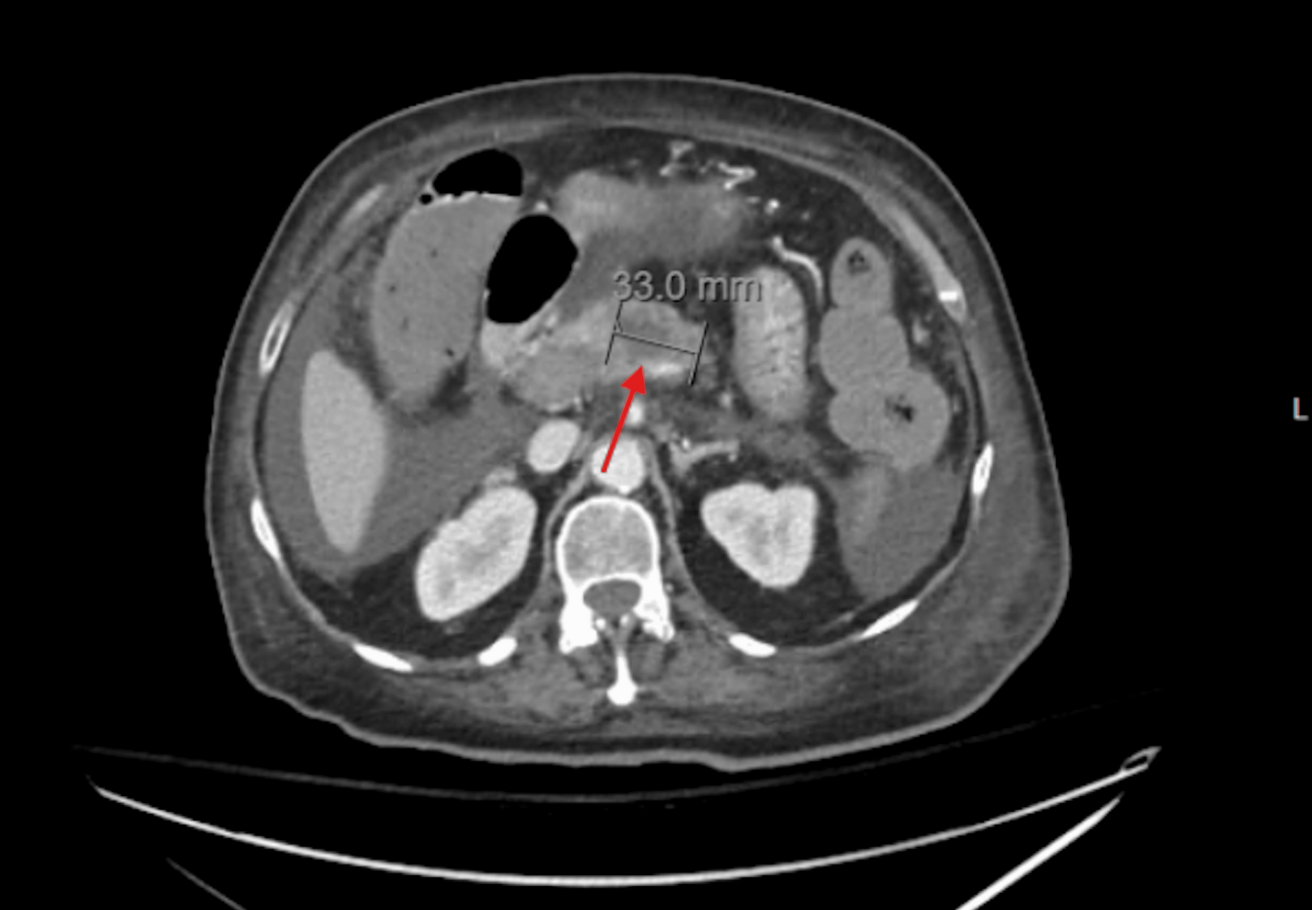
MRI image of hypoenhancing pancreatic mass in the proximal body of the pancreas. The arrow points to the mass and the line indicates its size.
